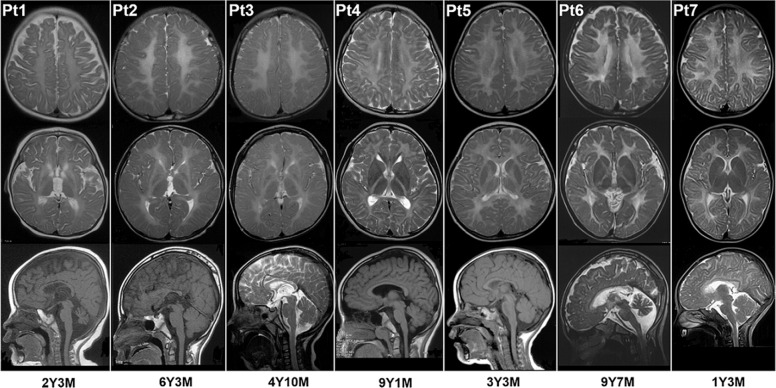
MRI images of seven patients. Row 1 and row 2 are axial T2-weighted images, showing diffuse hypomyelination in cerebral white matter in all seven patients and atrophy of the basal ganglia in Pt1, Pt2, and Pt3. Pt1 also demonstrated an abnormal signal in the putamen and caudate nucleus. Raw 3 are midsagittal T1-weighted or T2-weighted images, showing atrophy of cerebellum in Pt1 and Pt6. Six patients were diagnosed with HLD6 (Pt1, Pt2, Pt3), HLD7 (Pt4), HLD11 (Pt5, Pt6), and SOX10 related disorder (Pt7), respectively. Y years, M months
